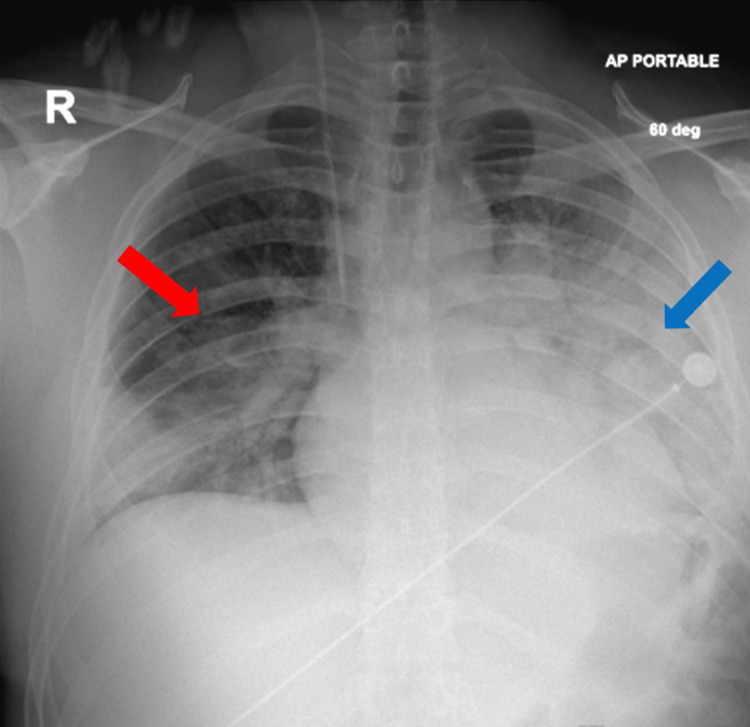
Chest x-ray Multifocal ground-glass infiltrates noted bilaterally (red and blue arrows) greater in the left lung (blue arrow) concerning for COVID-19 pneumonia COVID-19: coronavirus disease 2019
